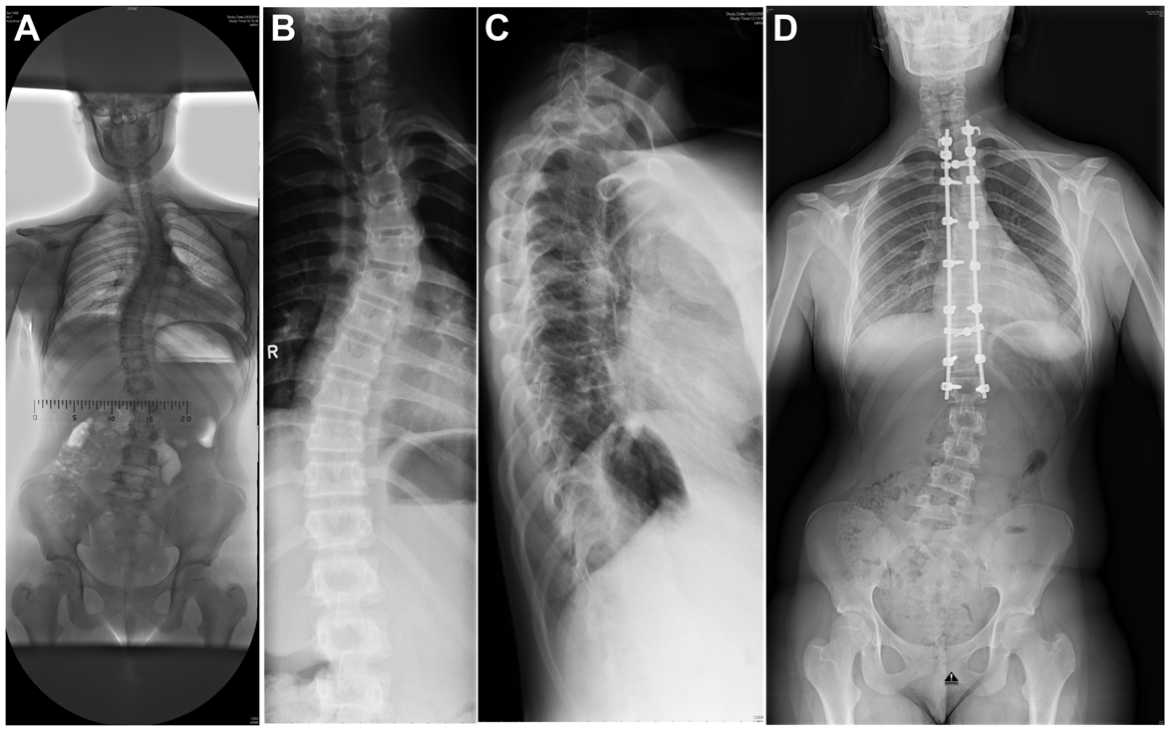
X-ray photographs of the thorax of the patient. A. Severe scoliosis. B. Close-up of the thoracic scoliosis. C. X-ray side view showing the kyphosis of the patient. D. X-ray of the thorax of the patient after corrective surgery for the progressive scoliosis.
